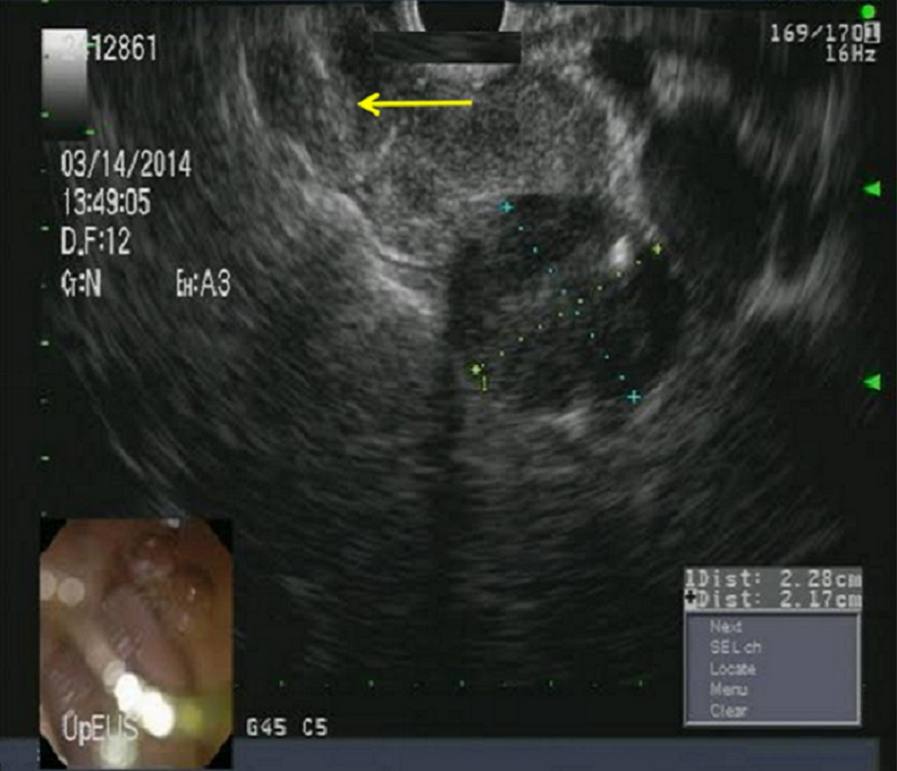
Endoscopic ultrasound Multiple enlarged peri-hepatic and peri-portal lymph nodes were identified on endoscopic ultrasound and a peri-hepatic lymph node was aspirated and biopsied (demarcated by the yellow arrow).
